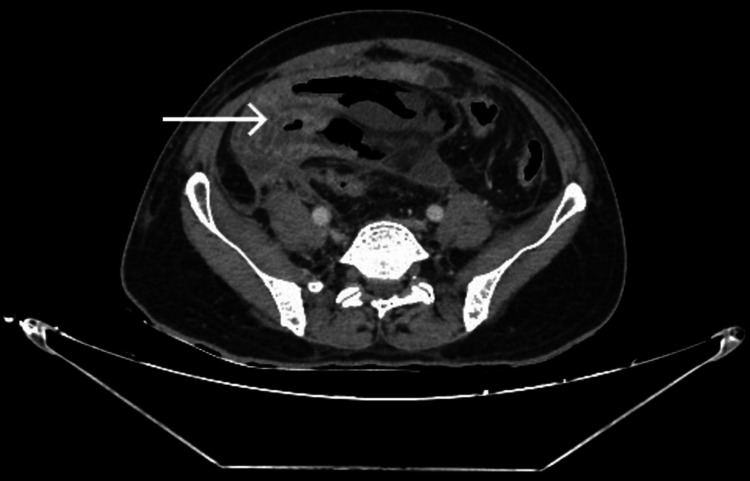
CECT abdomen axial view showing thickened ileal segments CECT: contrast enhanced computed tomography
